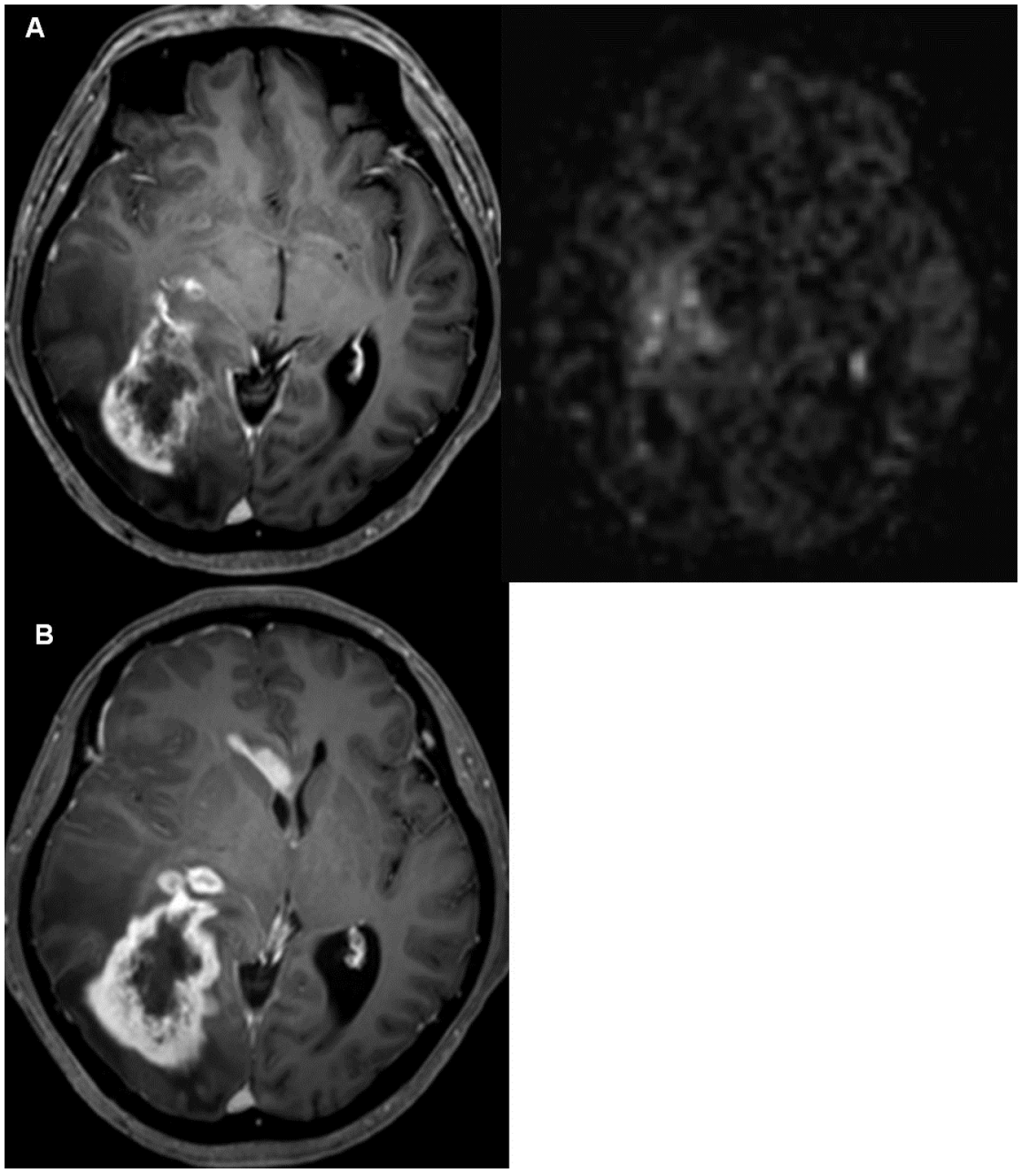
A*, MR images from a 63-year-old female patient with glioblastoma taken 2 weeks after concurrent chemo-radiation. The contrast-enhanced MRI (left) and ASL (right) showed negative perfusion in the surgical cavity wall enhancement (SCWE). *B*, Follow-up images taken 2 months later show evident tumour progression. Note that enhancing lesions increased mostly at the regions of previously negative perfusion, the posterior portion of SCWE.
